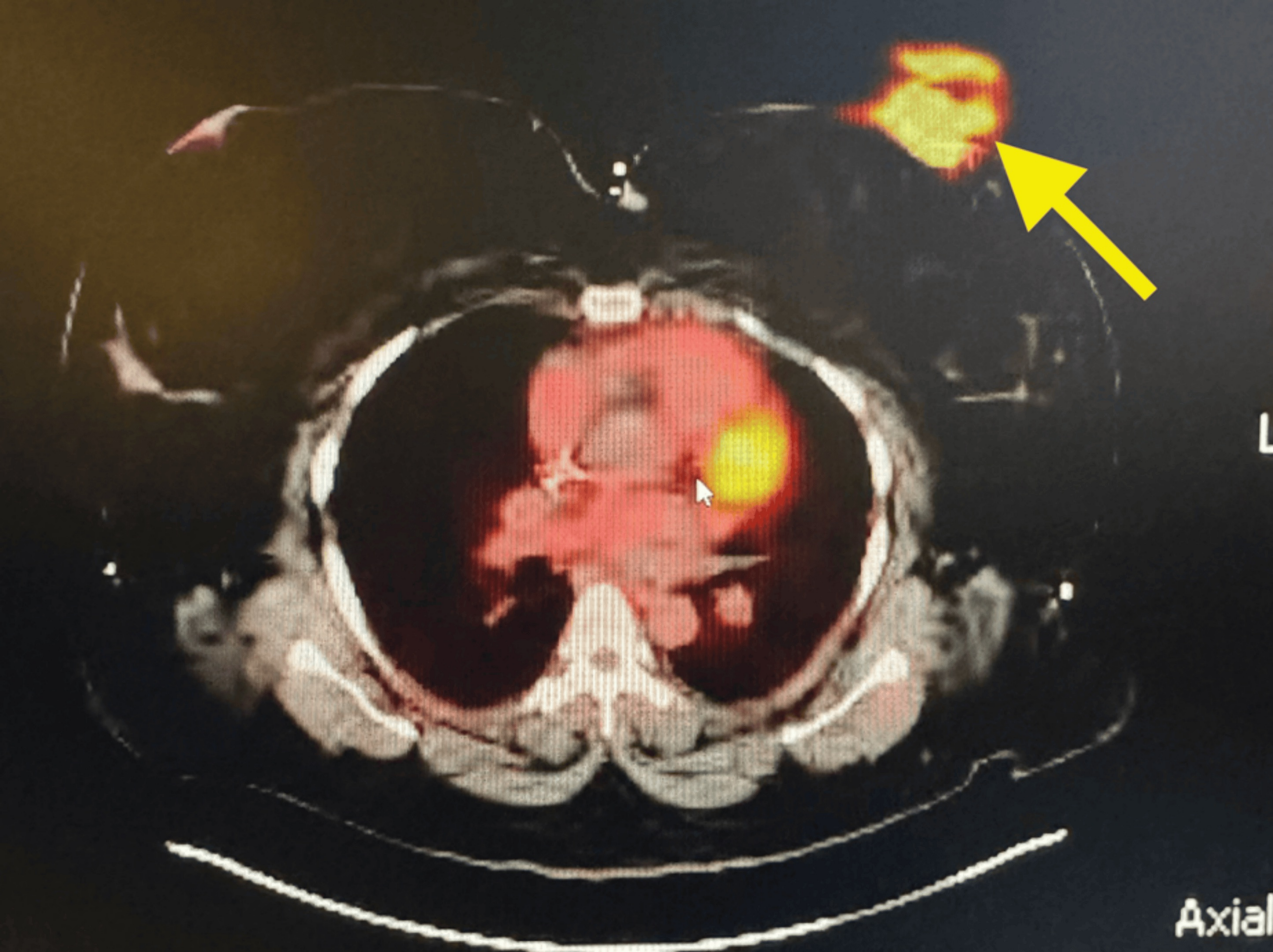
Axial PET/CT image demonstrating hyper-metabolic lesion in the left inner quadrant of the left breast (Slice 175/263) PET/CT: Positron Emission Tomography/Computed Tomography
